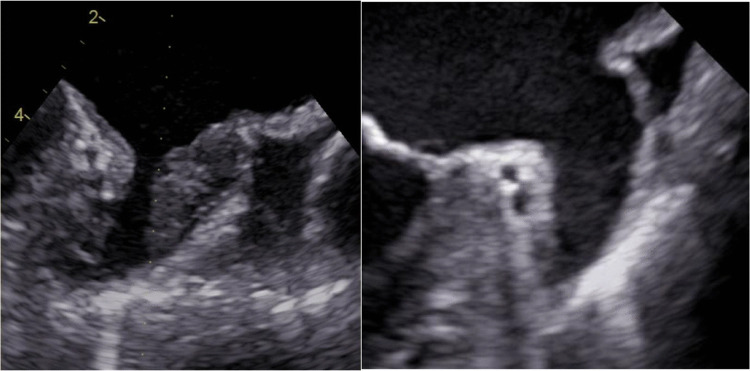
Identification of a solid thrombus occupying >50% of the LAA (“grade 3”). Transesophageal echocardiography eight weeks after showed thrombus resolution
